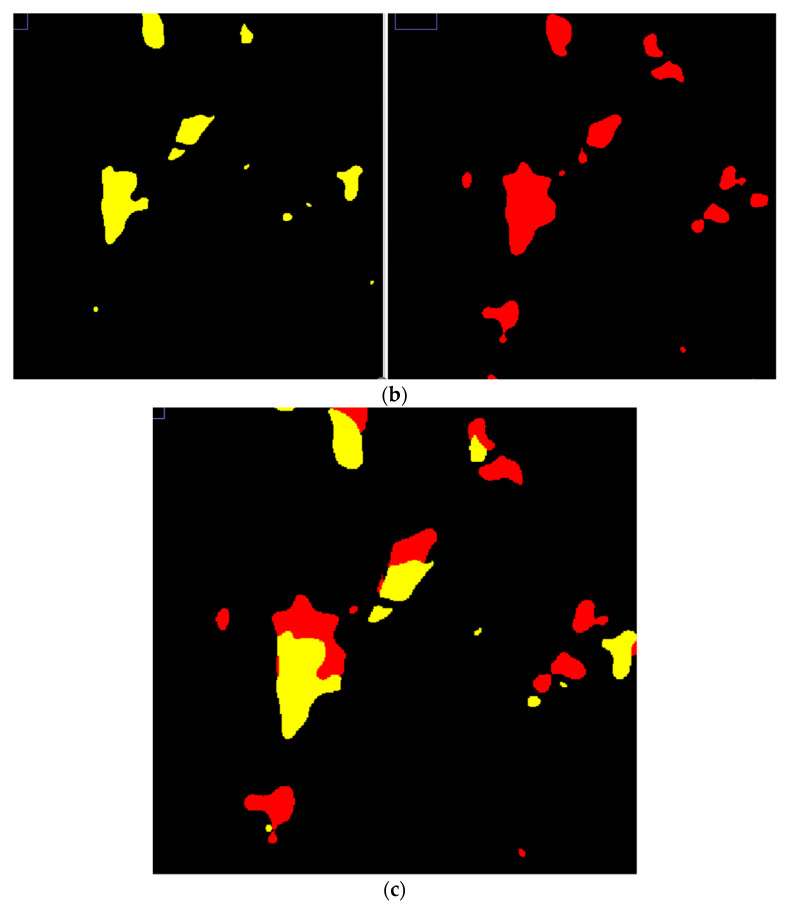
An example from Mix 1 showing the formation of new air voids and enlargement of the existing air voids after 90 F–T cycles: (a) X-ray CT scans for Mix 1 sample at 0 F–T cycles (left) and 90 F–T cycles (right); (b) ImageJ masks for Mix 1 sample at 0 F–T cycles (left) and 90 F–T cycles (right); (c) The original air voids at 0 F–T (yellow) with the new air voids (red) after 90 F–T.
